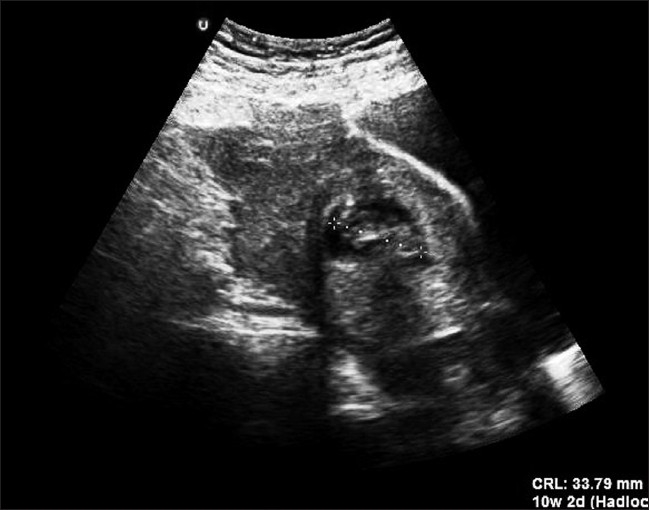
Ultrasonography showing free intraperitoneal fluid and a normal-looking intrauterine gestation with a positive fetal heart rate, consistent with a fetal age of approximately 10 weeks of amenorrhea
